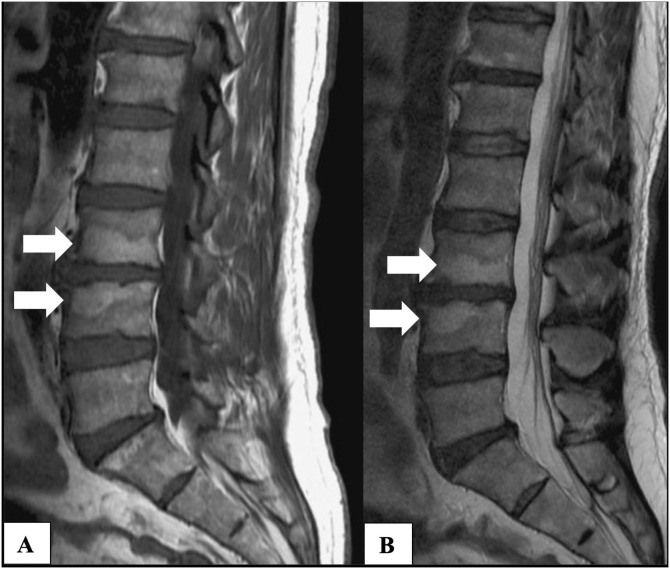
Type 2 Modic changes as visualized by magnetic resonance imaging. Sagittal T1-weighted (A) and T2-weighted (B) images of the lumbar spine showing hyperintense signal on T1 and isointense or mildly hyperintense signal on T2, indicative of fatty infiltration of the bone marrow at the vertebral endplates. These changes reflect a more chronic phase of degenerative disc disease compared to Type 1 Modic changes. White arrows identifying bone marrow with fatty replacement around the L3-L4 intervertebral space. (Image adapted from Modic Type II Endplate Changes by Frank Gaillard (https://radiopaedia.org/cases/modic-type-ii-endplate-change), Licensed under Creative Commons Attribution-ShareAlike 4.0 International Deed).
